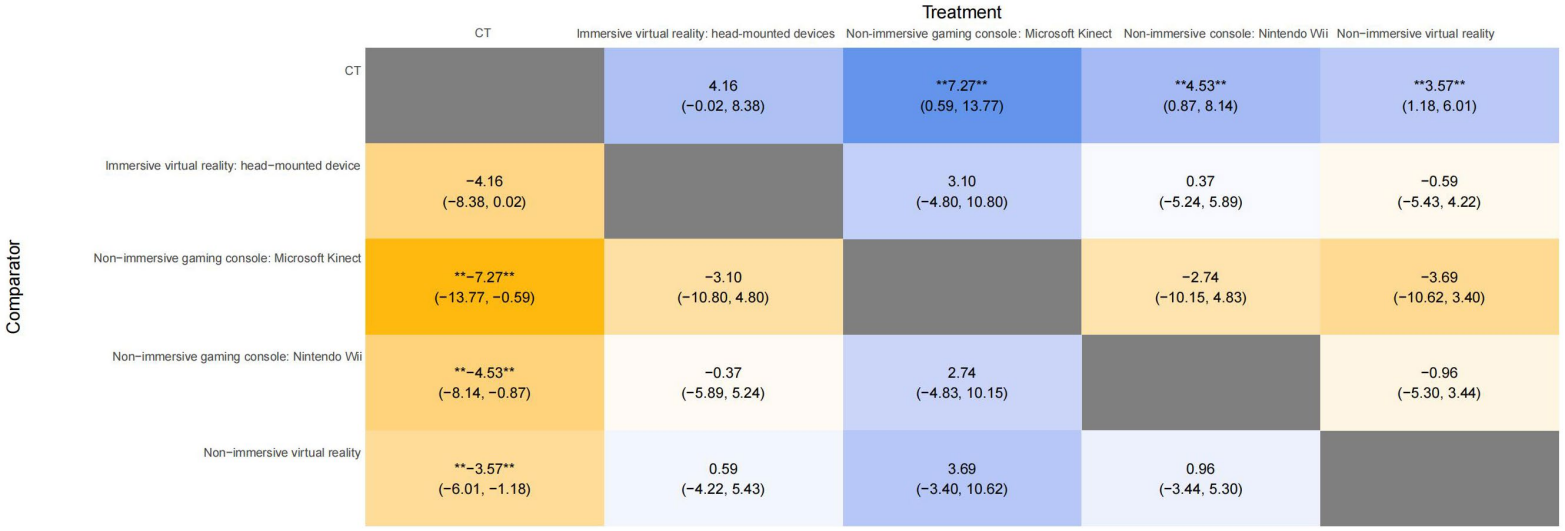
League table for all treatment in the network for FMUE. The symbol  in the figure indicates significant differences between the treatments (*p* < 0.05). FMUE, The Fugl-Meyer Upper Extremity; CT, Conventional therapy.
